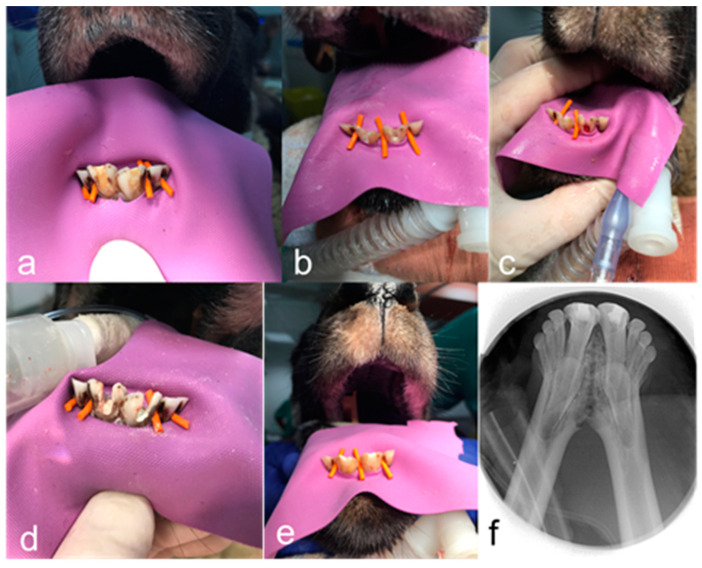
Representative clinical and radiographic images. (a) Preoperative image after rubber dam isolation of teeth 31 and 41. (b) Visualization of calcium hydroxide after access opening. (c) Induction of bleeding in the root canal of tooth 41. (d) Placement of intracoronal sealing material: Biodentine in tooth 31 and ProRoot White MTA in tooth 41. (e) Restorative build-up of teeth 31 and 41 after revitalisation therapy. (f) Postoperative radiographic image of teeth 31 and 41. Phase 4.
